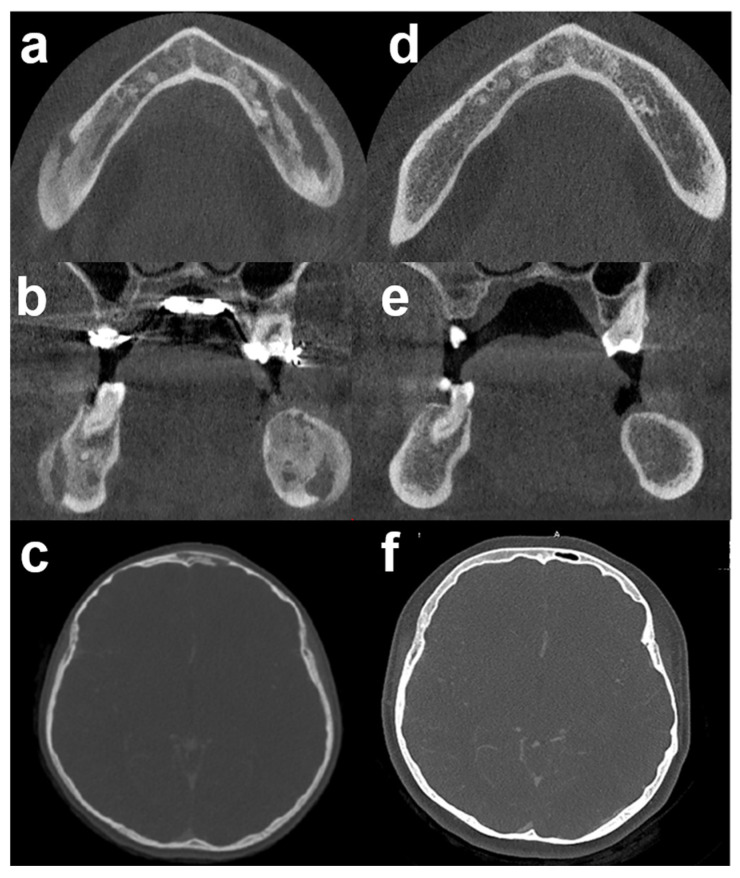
3D computed tomography before and after antibiotic therapy: (a–c) CBCT/CT scan before antibiotic therapy. (d–f) The corresponding CBCT/CT scan, 6 months after antibiotic therapy demonstrated complete bone healing. Bone sclerosis and osteolysis (mixt pattern) disappeared in mandible (d,e) and frontal bone (f). CBCT: Cone Beam Computed Tomography.
